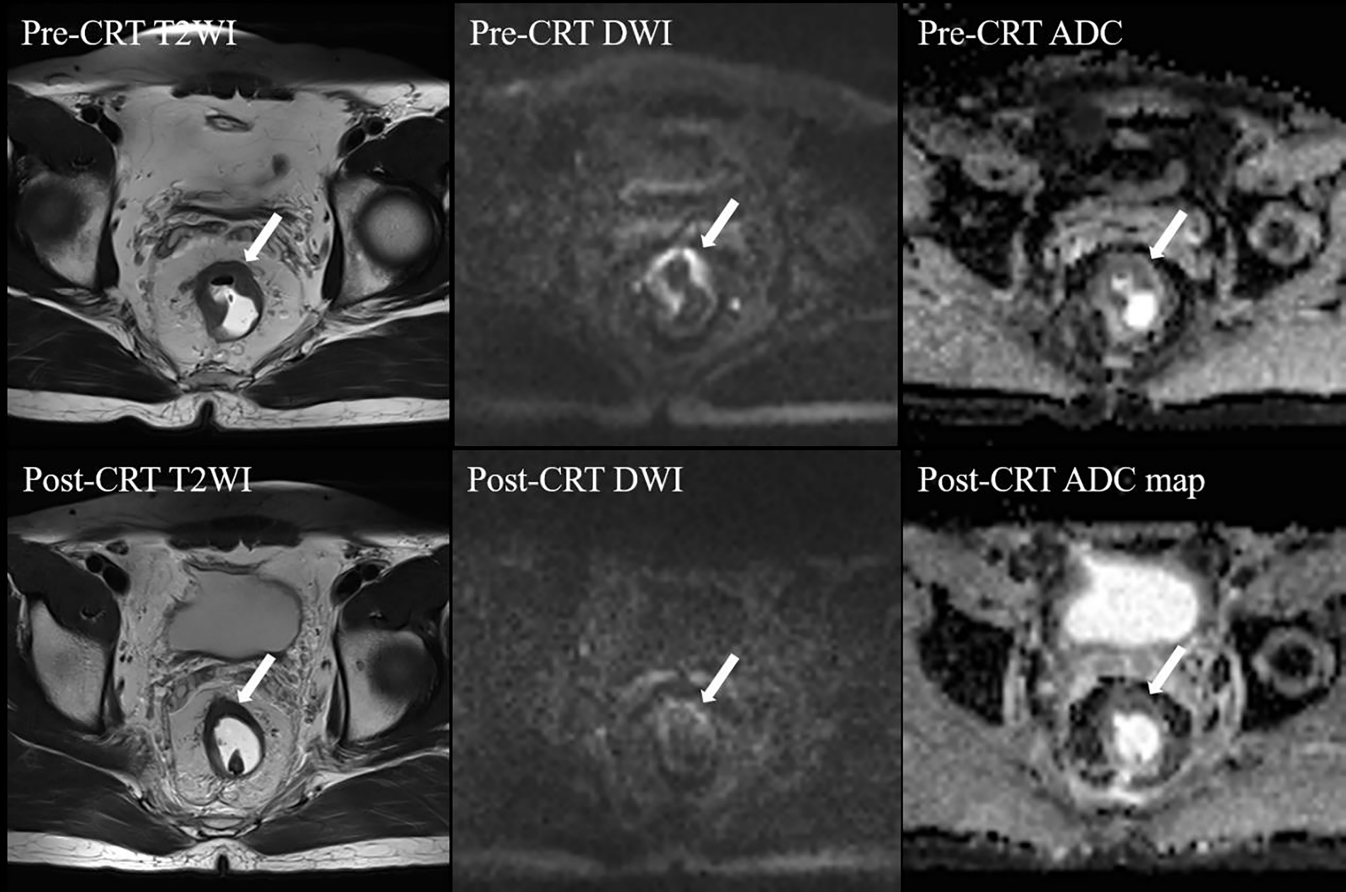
MRI tumor regression grade 2 in a 43-year-old man after neo-CRT. Post-neo-CRT T2WI shows a remarkable decrease in the tumor size with the remaining hypointense “fibrotic” thickening of the wall without visible tumor signal, whereas high *b* value post-CRT-DWI shows linear high signal intensity at the tumor bed, with low signal intensity on post-CRT-ADC map, indicating residual tumor. CRT, chemoradiotherapy; T2WI, T2-weighed imaging; DWI, diffusion-weighted imaging; ADC, apparent diffusion coefficient. The white arrows indicate tumor.
